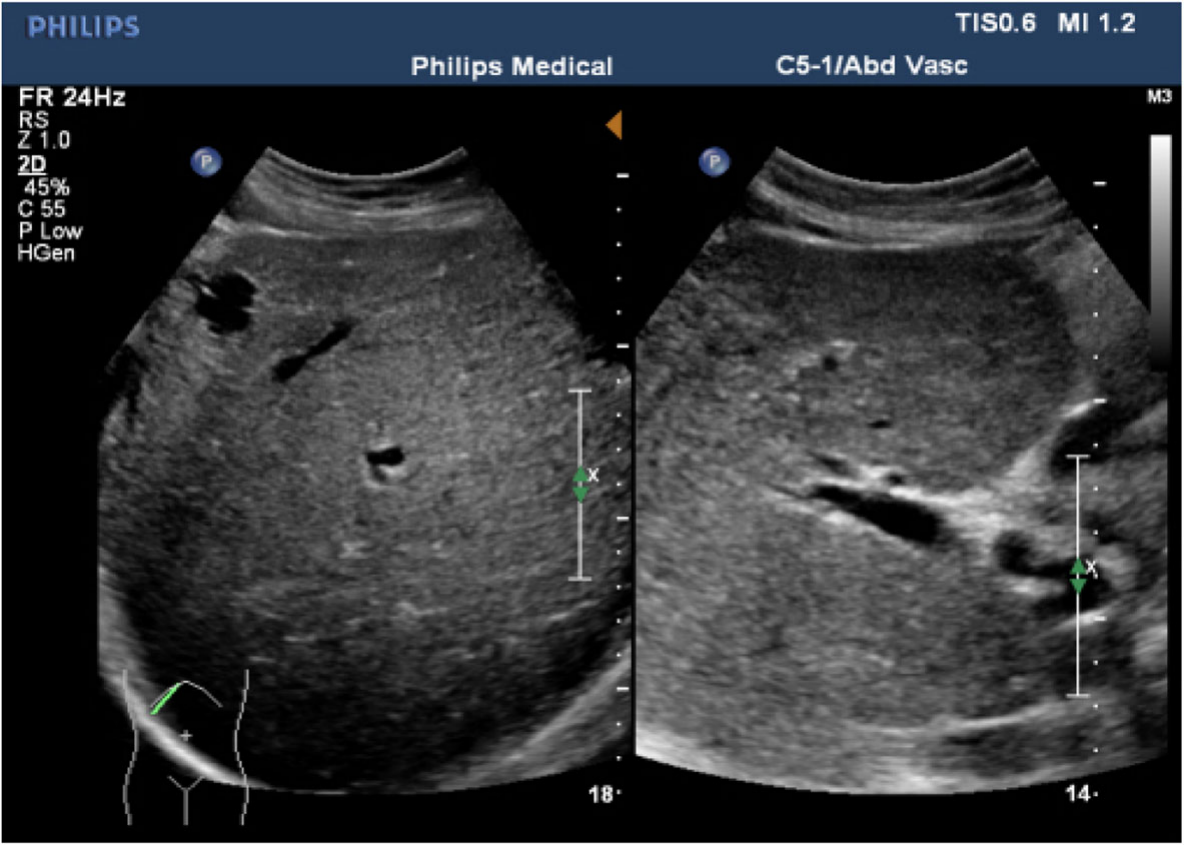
B-ultrasound of the abdomen: Ultrasound shows the left hepatic vein to be narrow (~ 0.5 cm in inner diameter). The right hepatic vein is narrow (the inner diameter is ~ 0.38 cm). The middle hepatic vein is occluded (inner diameter is ~ 0.38 cm; the distal end is dilated, with an inner diameter of ~ 0.8 cm)
